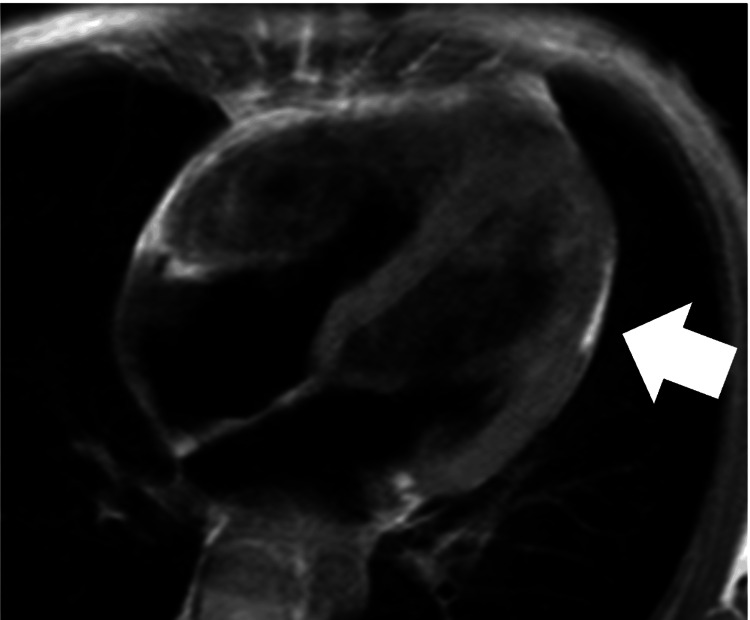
Cardiac magnetic resonance imaging of case 3 at diagnosis T1-weighted magnetic resonance imaging showing fat deposit from anterolateral to the apical wall of the left ventricle
